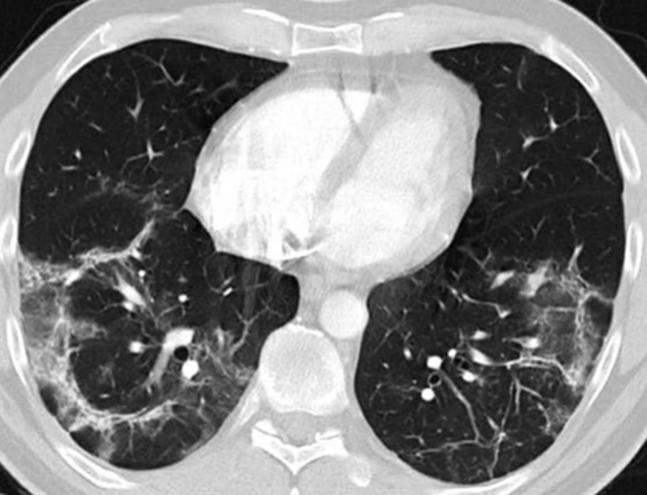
Lung CT scan in the lower lobes showing ground glass, arcade-like bands of parenchymal consolidation, peribronchial consolidation and mild bronchiolectasis
